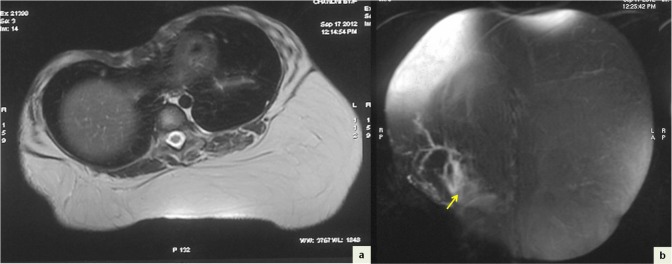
Figure 1: Axial T2-weighted (a) and coronal fat-saturated (STIR) images (b) showing a large lobulated predominantly fat intensity lesion with multiple septae within the subcutaneous tissue of back. A soft tissue component is seen along its right inferolateral aspect (arrow in b).
